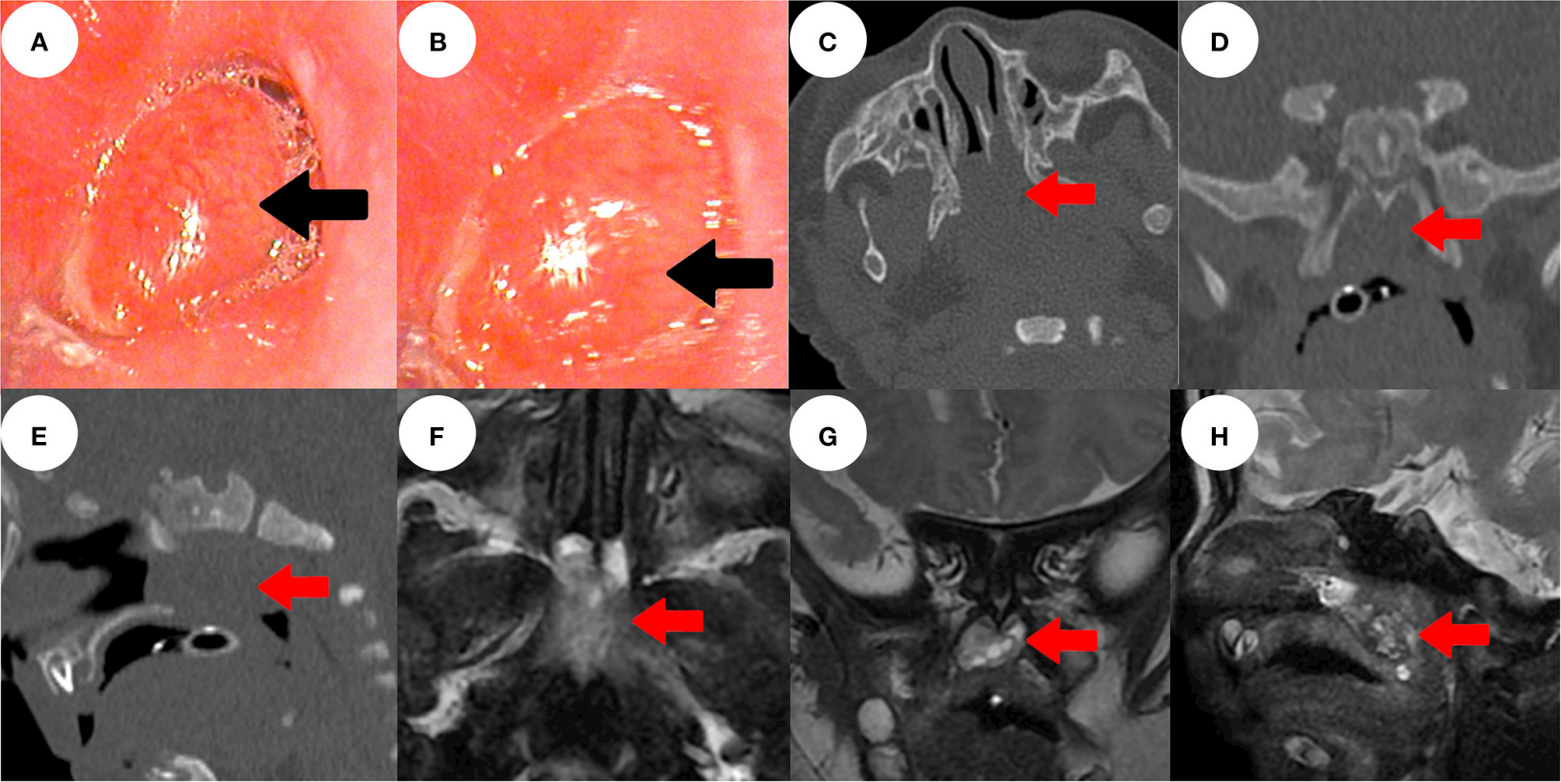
Electronic nasopharyngoscopy showed a red-pink smooth mass with an indistinct base, which almost completely blocked the posterior nostrils (black arrow) bilaterally (A,B). Sinus computed tomography showed a mass with irregular soft tissue density in the nasopharynx (red arrow) (C–E). Sinus magnetic resonance imaging revealed that the nasopharyngeal mass had irregular mixed signals (F–H).
